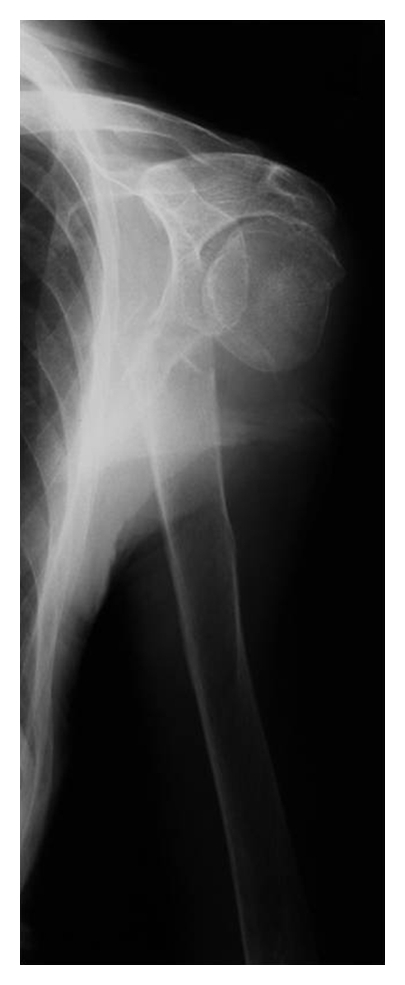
Initial X-rays of the dislocated proximal humeral fracture (Scapula-Y-view).
